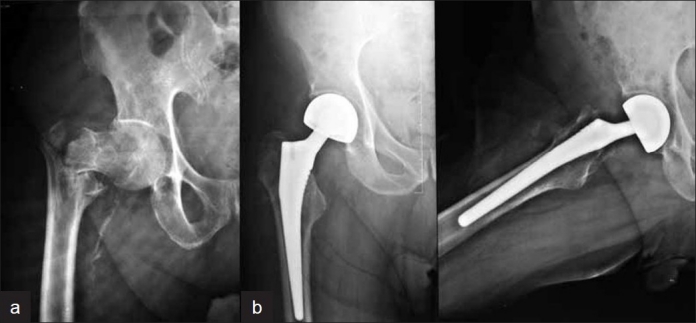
Preoperative radiographs of right hip (anteroposterior view) of 73-year-old patient shows (a) unstable intertrochanteric fracture of the femur; (b) radiograph (anteroposterior and frog leg view) 24 months after cementless bipolar hemi-replacement
